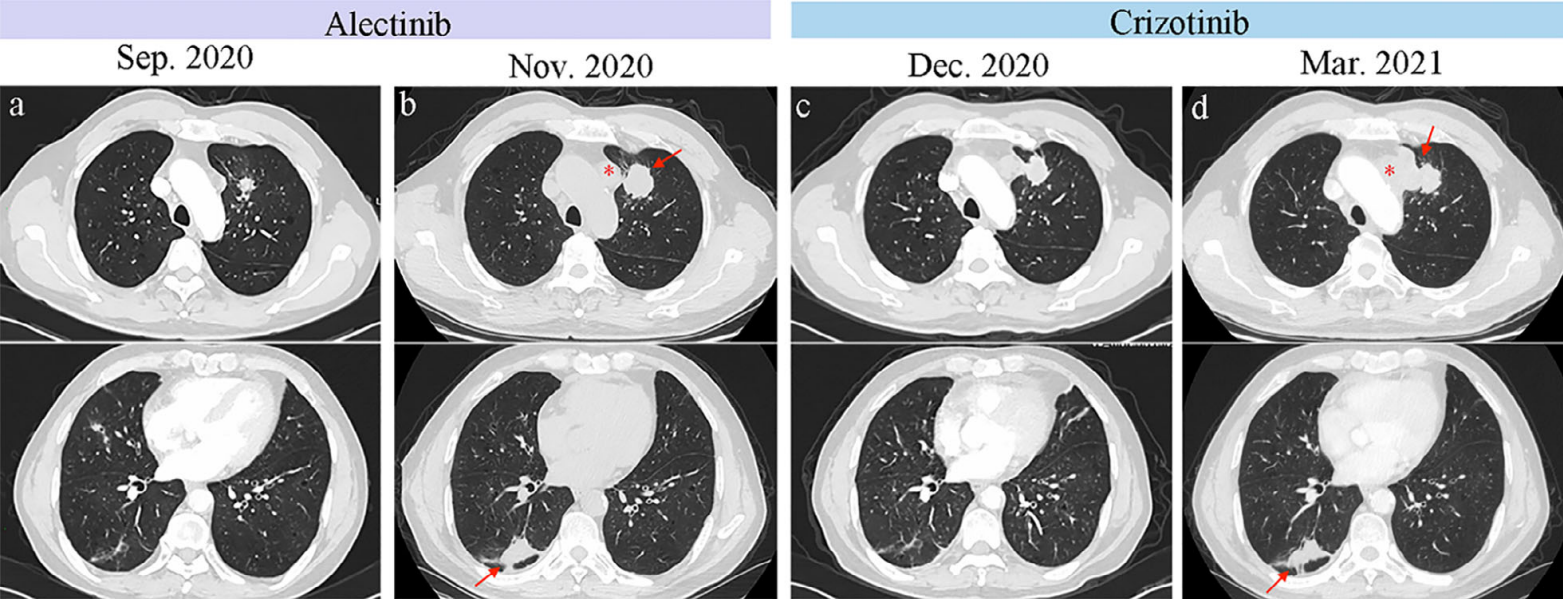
Images of chest computed tomography (CT) scans of the patient during the course of treatment. (a) Computed tomography scans during initial diagnosis. (b) One and a half months after initiation of alectinib. (c) One month after initiation of crizotinib. (d) Four months after crizotinib, the CT scan shows enlarged lymph nodes (asterisk) and an increase in size of the lung masses (arrow)
